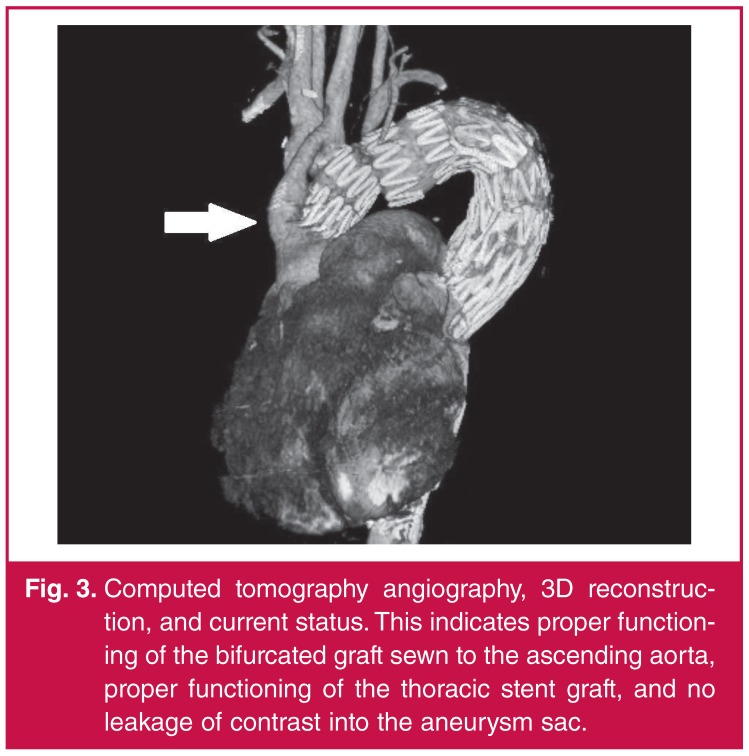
Computed tomography angiography, 3D reconstruction, and current status. This indicates proper functioning of the bifurcated graft sewn to the ascending aorta, proper functioning of the thoracic stent graft, and no leakage of contrast into the aneurysm sac.
